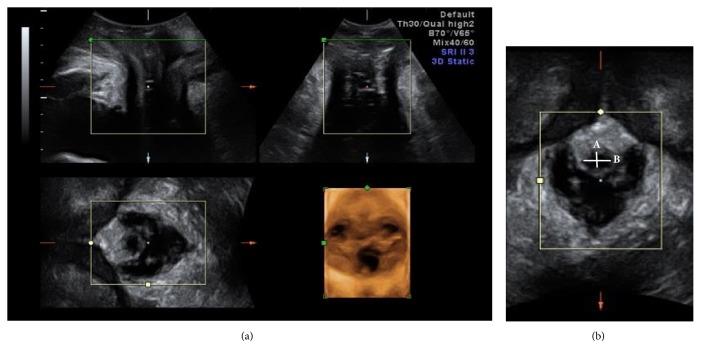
(a) Perineal sonography. Lower urinary tract on three-dimensional transperineal ultrasound imaging in a woman. The top left image shows the mid-sagittal view (A-plane). The top right image is a coronal view (B-plane); the bottom left is the axial or C-plane, showing hypoechogenic area of urethra. The bottom right image is the resulting rendered volume. (b) Axial view of the urethral hypoechoic core. Ultrasound image (axial plane) of the mid-urethral hypoechoic core in a woman with SUI, showing the measurements made for this study: the shortest (A) and longest (B) diameters of the urethral hypoechoic core.
